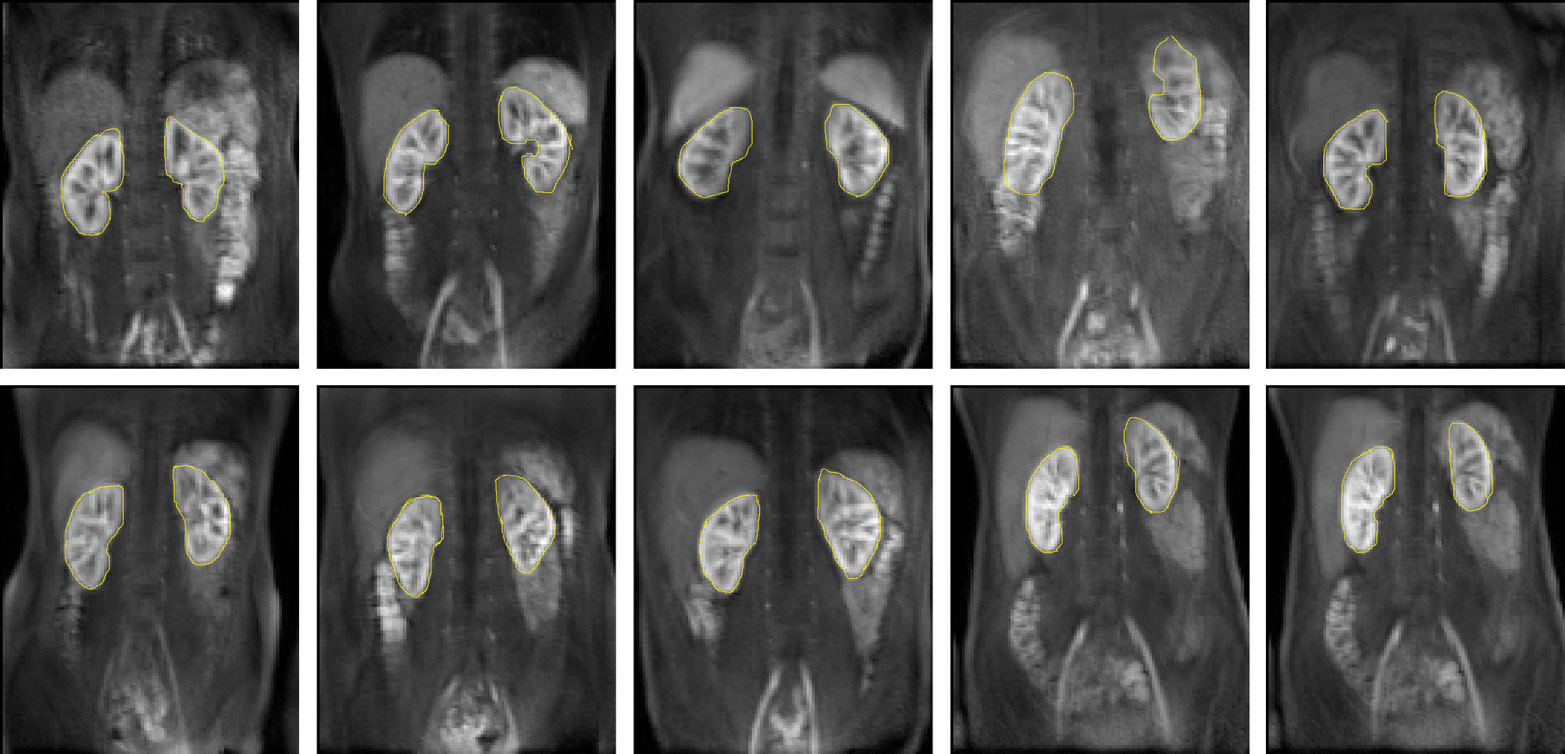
Exemplars of dynamic MR images from 10 healthy volunteers’ kidney slice produced by DCE-MRI sequence considered as 10 different data sets in this study. The images here show the central kidney slice at time 120*s* aortic peak enhancement after the contrast agent is injected. The *yellow* boundary on the kidneys is a result of manual delineation from a human expert. The mean intensity values are calculated in this region across the time producing time–intensity plots (colour figure online)
